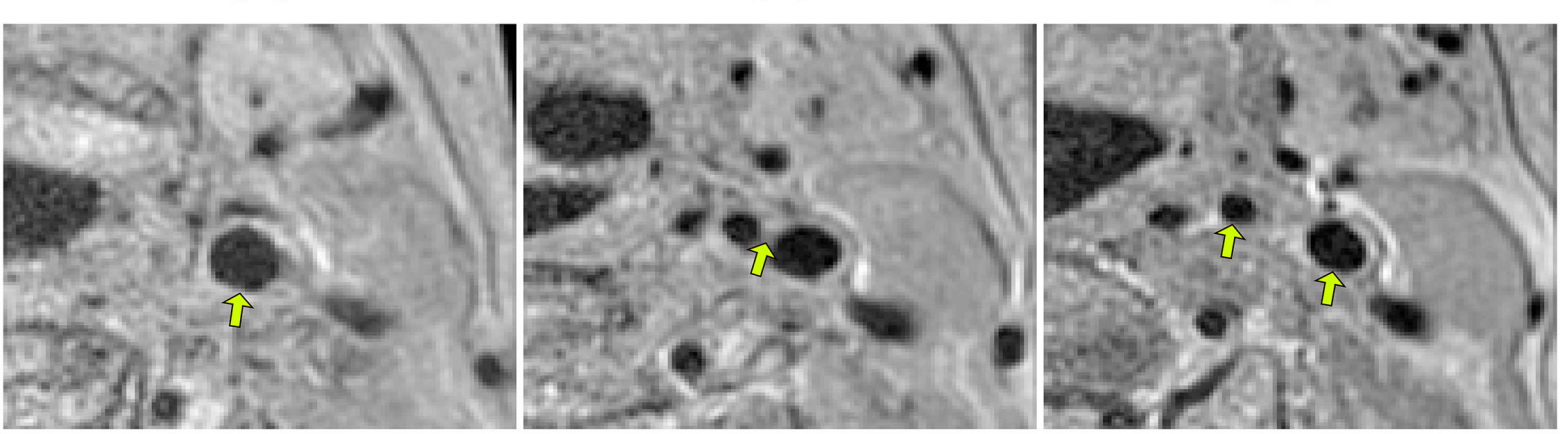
Carotid artery MRI. Cropped slices 10, 20, and 30 (left to right) of the left carotid of one subject in the carotid MRI data set. The arrows point to the common carotid artery (left image), the carotid bifurcation point (center image), and the internal and external parts of the carotid after the bifurcation (right image).
